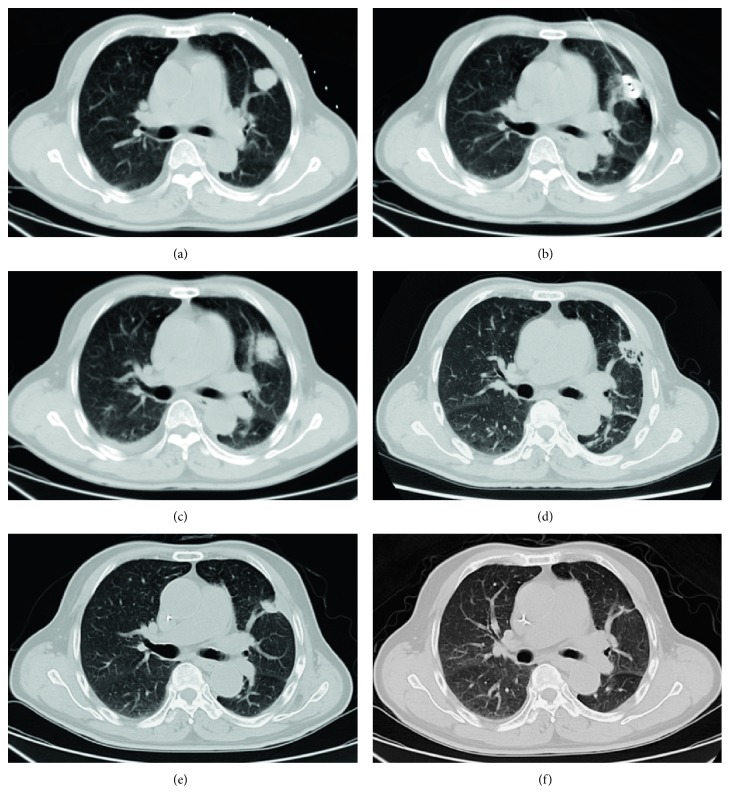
A 73-year-old man with lung metastasis from colorectal carcinoma in left upper lobe with an axial diameter of 2.3 cm (a). Ablation was performed after accurate insertion of MWA probe into the focus of tumor (b). The immediate postoperative CT scan showed that the lesion enlarged slightly with ill-defined and irregular margin (c). The lesion was shrunk gradually and replaced by fibrous tissue during 1 (d), 3 (e), and 6 (f) months follow-up.
